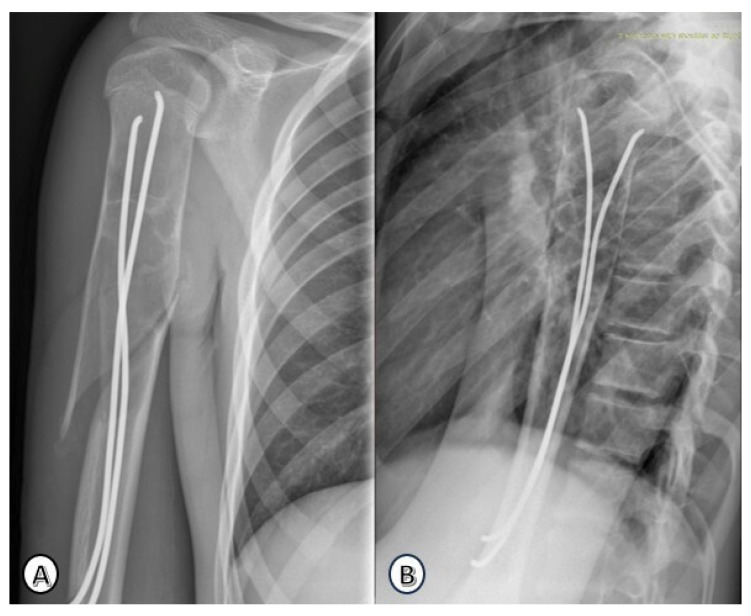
Postoperative radiographs of the same patient after intramedullary stabilization using a 3 mm titanium elastic nail. (A): Postopertaive anteroposterior view, (B): Postopertaive lateral view.
